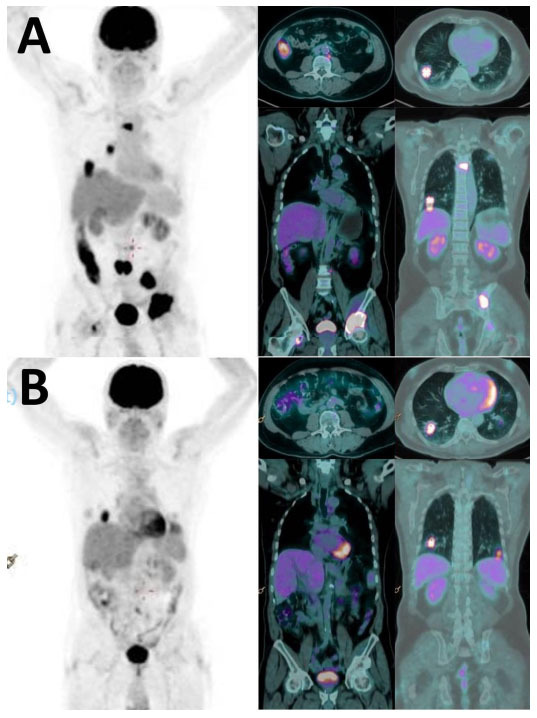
Baseline and follow-up PET-CT. (A) PET-CT/18F-FDG before immunotherapy showing the primary lung lesion and metastases, with the most prominent in the left hip (February 2019). (B) Follow-up PET-CT/18F-FDG showing mild lung lesion regression, fewer active metastatic sites, and an increment in myocardial 18F-FDG uptake (October 2019).
